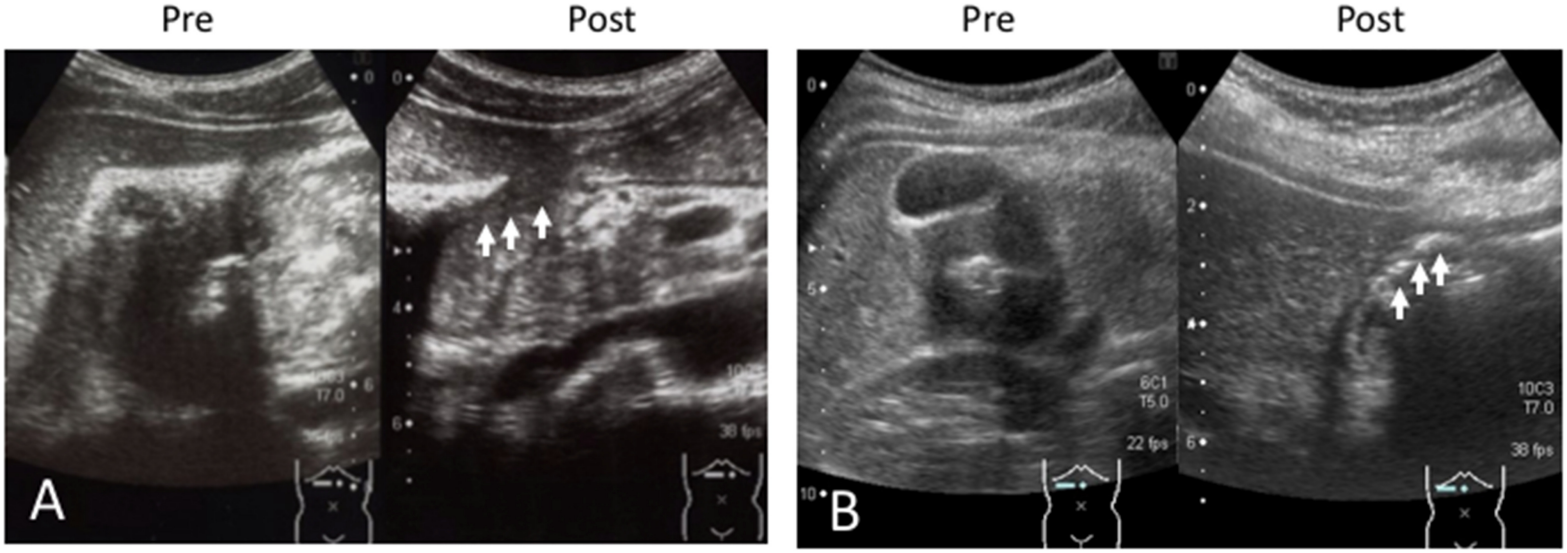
(A) Case 2 and (B) Case 3. The sonographic images in the recurrent episode are shown. The hypertrophic wall and echogenic line (HH sign) are shown in “Pre.” The HH sign diminished on treatment with the antiulcer drug (arrows) as shown in “Post.” Pre, pretreatment; Post, post-treatment with antiulcer agent.
